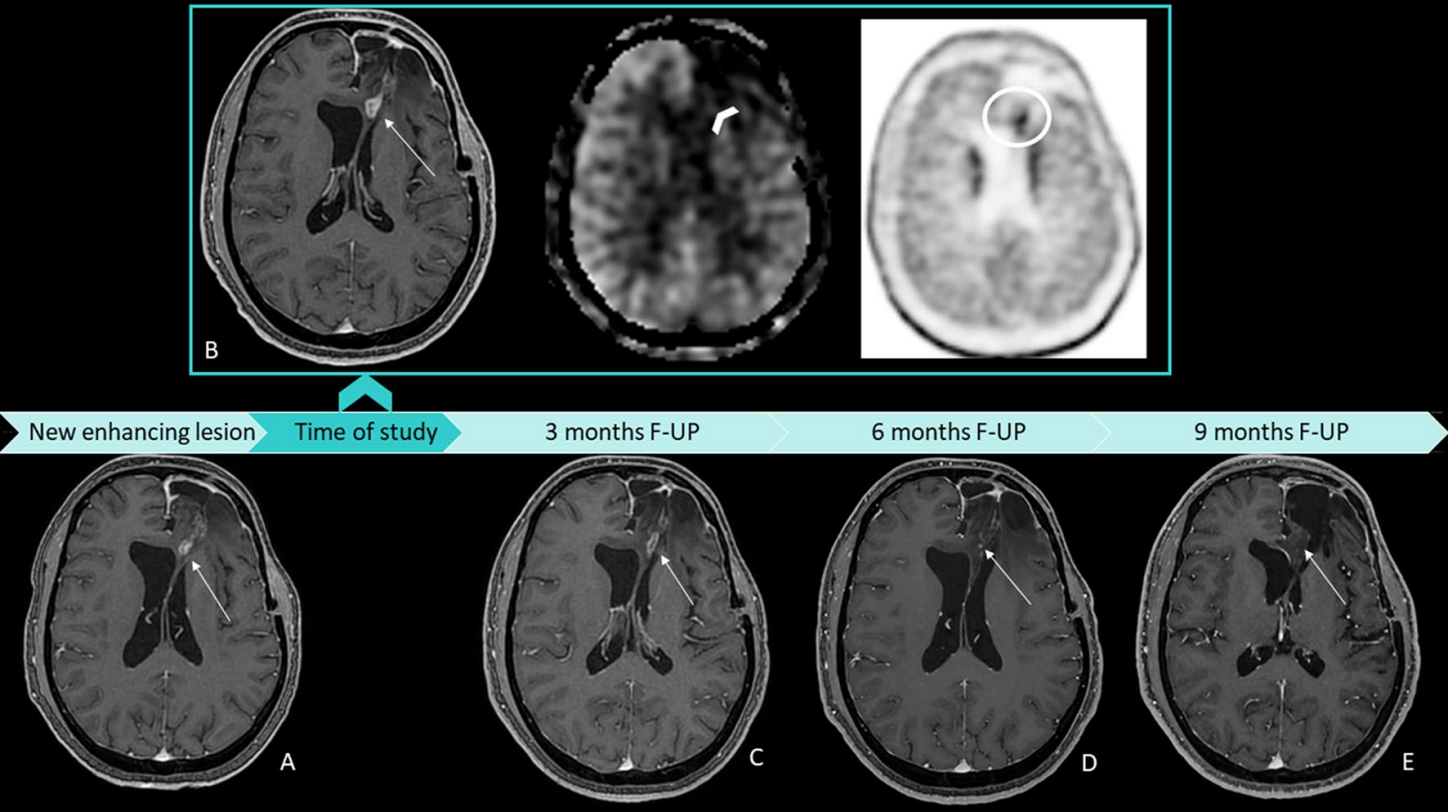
A representative case of high-grade glioma with a new enhancing lesion appeared 3 months after radiation therapy (a, arrow); the patient was included in our study, and after 2 months he underwent MRI and PET examination following study protocol (b, squared images). On T1 post-contrast WI, the lesion was increased in size (b, arrow), the ASL_CBF map showed no increment of CBF (b, arrowhead), and 18F-DOPA PET/TC showed an increased uptake (b, circle). T1 post-contrast WI acquired, respectively, after 3 (c) and 6 (d) months, showed a progressive reduction of contrast enhancement and lesion size until, after 9 months (e), the lesion was no longer present. This is a case of pseudoprogression with a false positive PET/CT and a true negative ASL classification. ASL = arterial spin labeling; CBF = cerebral blood flow; DSC = dynamic susceptibility contrast-enhanced perfusion; DCE = dynamic contrast-enhanced perfusion; CBV = cerebral blood volume; Ve = fractional volume of the extravascular extracellular space; WI = weighted imaging
